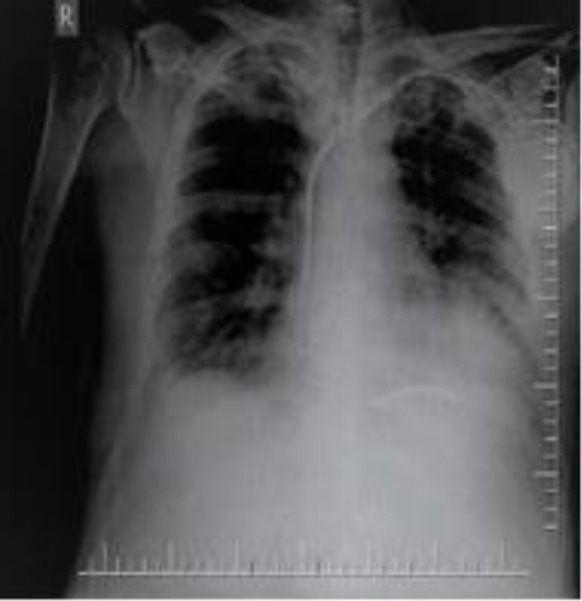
Implantable cardioverter defibrillator, increased cardiothoracic ratio, bilateral pleural effusions, and dual chamber pacemaker on chest x-ray.
